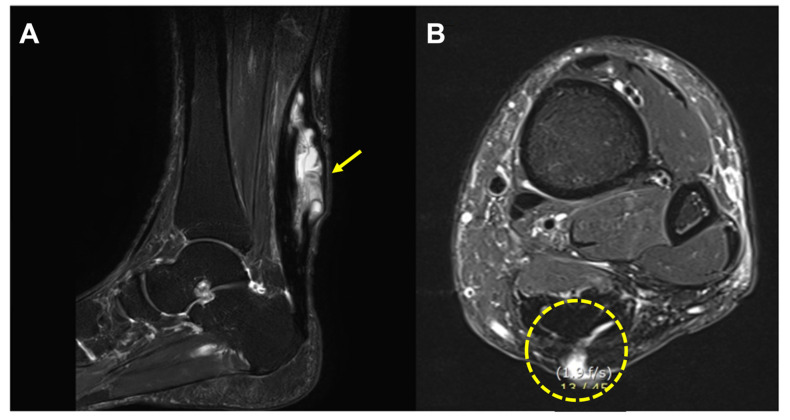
Magnetic resonance imaging (MRI) images. (A) A high-signal irregularly walled cystic structure (arrow) within the thickened Achilles tendon substance (patient 7). (B) The lesion communicated with subcutaneous space and overlying skin through draining sinuses (dotted circle).
